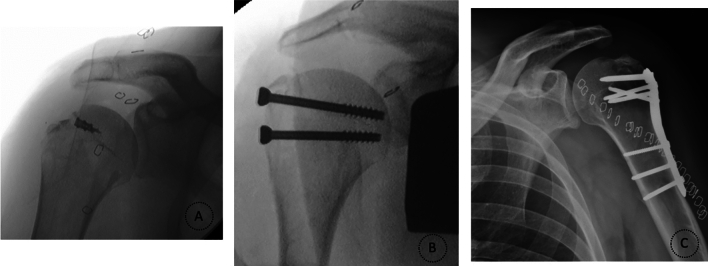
Radiographs showing surgical management of the greater tuberosity fractures in the proximal humerus. A Anchor repair; B Interfragmentary screws; C Locking plate fixation
